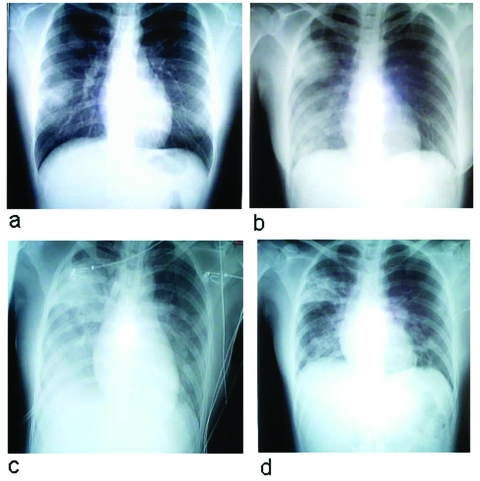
Chest radiographs of index patient with severe acute respiratory syndrome (SARS). a, day 5 of symptoms; b, day 10; c, day 13; d, day 15.
